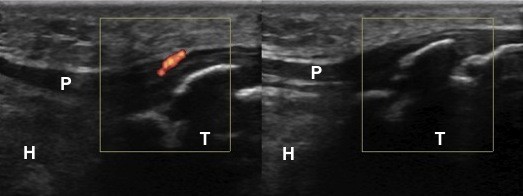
A 10-year-old girl with unilateral knee pain who was eventually diagnosed as bilateral Osgood-Schlatter disease and patellar tendinitis on the symptomatic side. Comparative power Doppler ultrasound evaluations (longitudinal view) demonstrate cortical irregularities at the insertion site of the patellar tendon (P) on the tibia (T) bilaterally. There is also abnormal power Doppler signal on the patellar tendon (left image). The Hoffa's fat pad (H) is normal on both sides.
